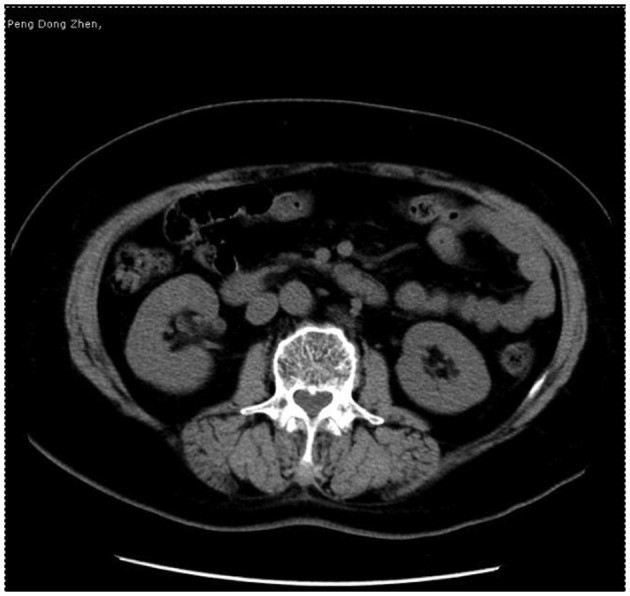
Case 1. CT plain scan shows the disappearance of the low density focus and the iliopsoas abscess. CT, computed tomography.
